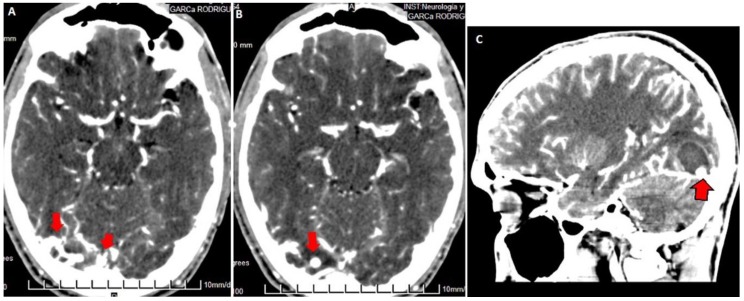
Contrasted brain CT: in axial section (A,B) dilated vessels are observed in the right occipital region (red arrows in (A)) with saccular venous ectasia (red arrows in (B,C)). Sagittal reconstruction (C) shows intraparenchymal hematoma in resolution with a ring-like enhancement on its periphery. The dilated saccular vein is in contact with the lower edge of the hematoma (red arrow with a black border).
